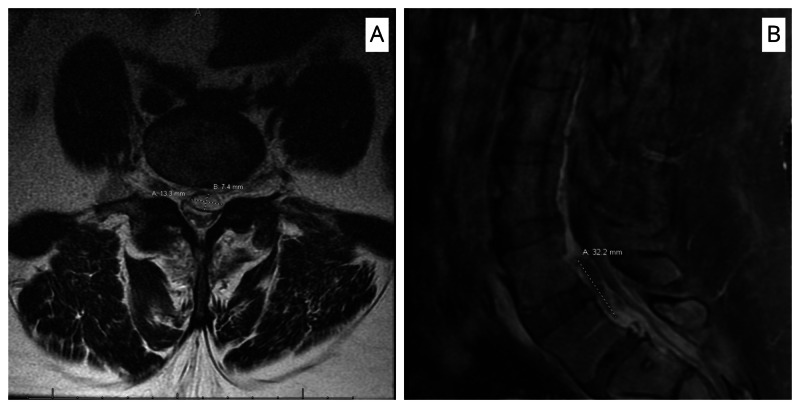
MRI scan (T1 with contrast) of the spine showing epidural abscess measuring 32.2 × 13.3 × 7.4 mm posterior to L4-L5 vertebral body with compression of thecal sac. (A) Axial section. (B) Sagittal section.
